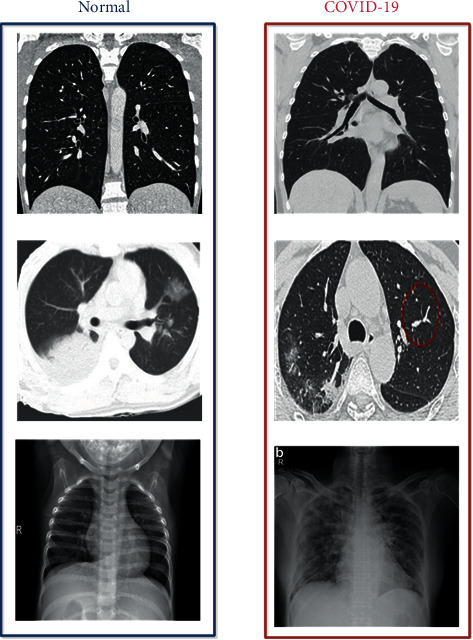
Example of X-ray and CT images of a normal patient and a COVID-19 patient.
